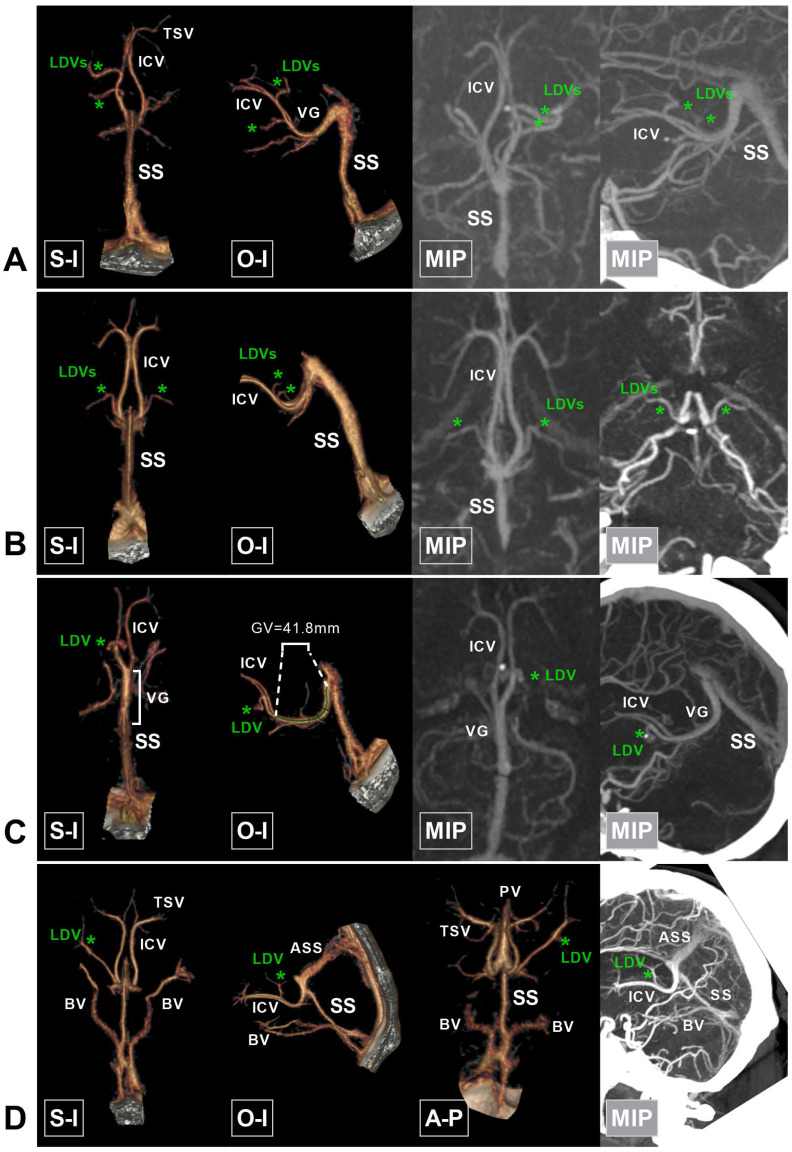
Rare variations in the deep cerebral veins around the VG. A, CTA and MIP show duplicate LDVs (asterisk) draining into the left ICV. B, CTA and MIP show that the LDVs (asterisk) drain into the terminal part of the bilateral ICVs. C, CTA and MIP show a very long VG (41.8 mm) and that the bilateral ICVs merge early. D, An accessory SS (ASS) is noted above the SS, which has the same draining function as the SS. The SS decreases accordingly. The bilateral BVs drain directly into the terminal portion of the SS. Abbreviations: A-P, anterior to posterior; ASS, accessory straight sinus; BV, basal vein; CTA, computed tomography angiography; ICV, internal cerebral vein; MIP, maximum intensity projection; O-I, outside to inside; S-I, superior to inferior; SS, straight sinus; TSV, thalamostriate vein; VG, vein of Galen.
